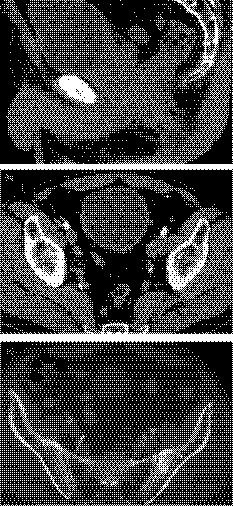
Pelvic computed tomography at diagnosis. (a) Sagittal view shows that the tumor has invaded the bladder. (b, c) Horizontal views show metastases to the bilateral lymph nodes and iliac bone.
